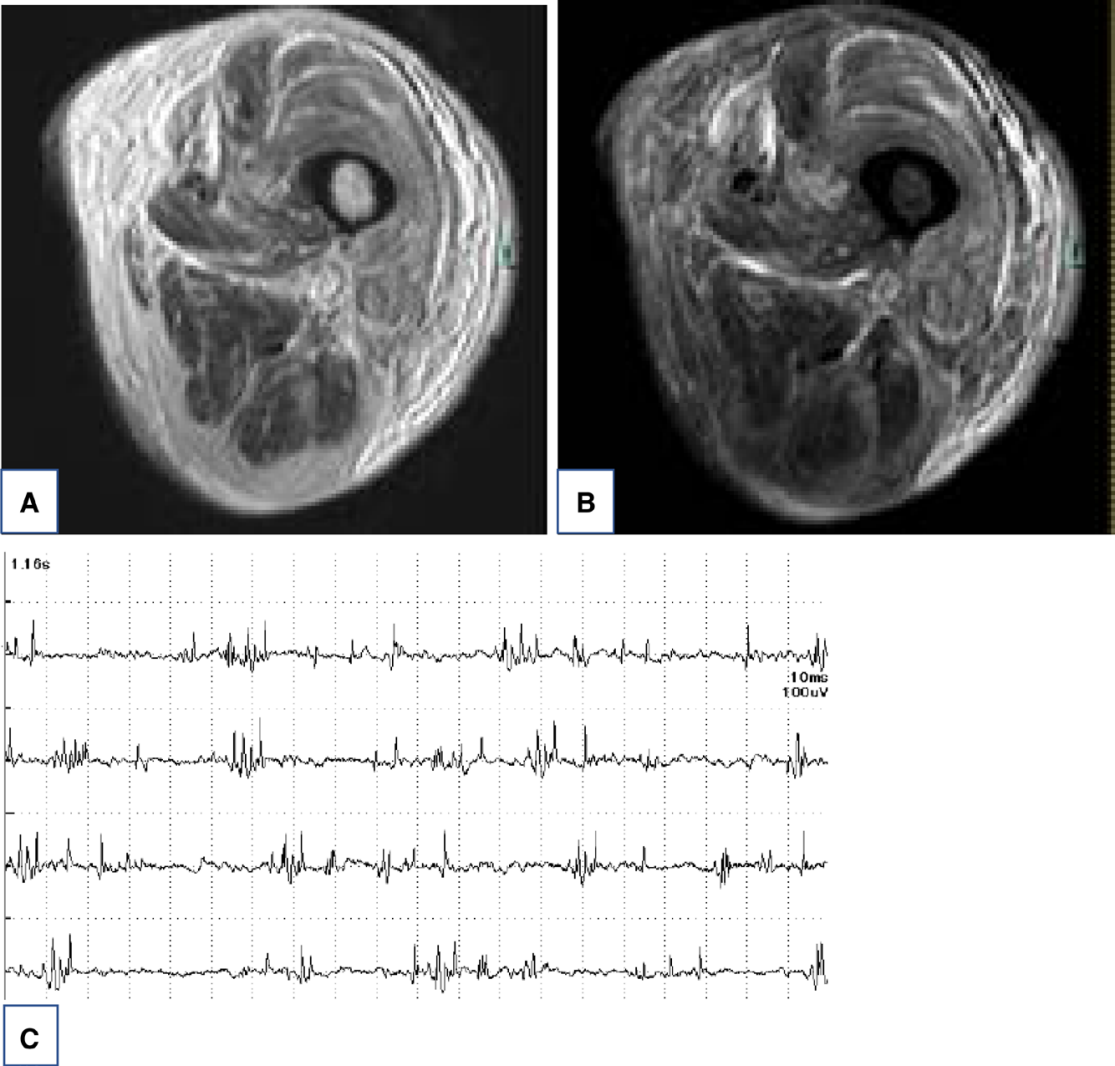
Magnetic resonance imaging of the left thigh showed a high signal intensity in T2-weighted and (A) short TI inversion recovery images (B). The needle electromyogram shows early recruitment and motor unit potential with low amplitude and polyphase (C).
